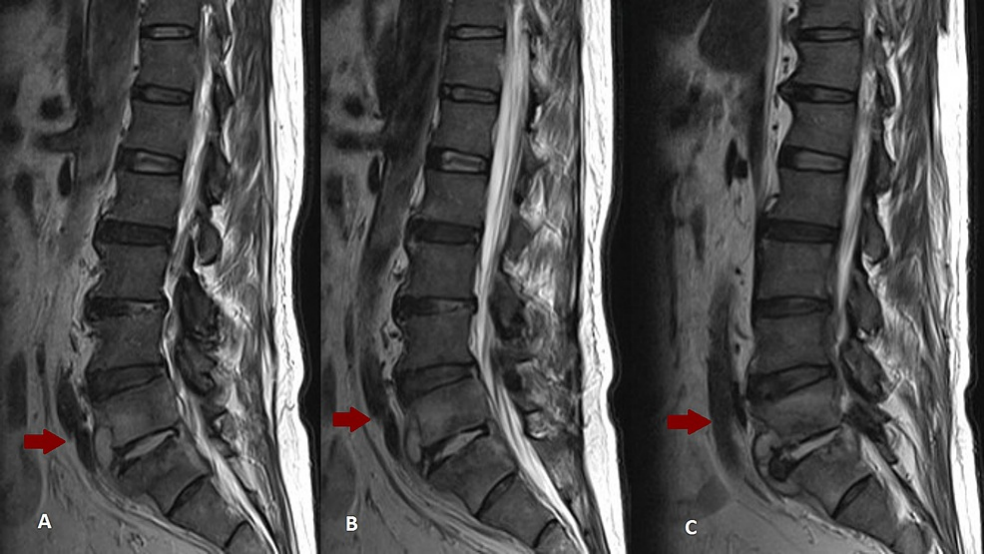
Lumbar spine MRI scan. (A-C) Multiple sagittal cuts of L5-S1 spondylodiscitis. MRI - Magnetic resonance imaging
